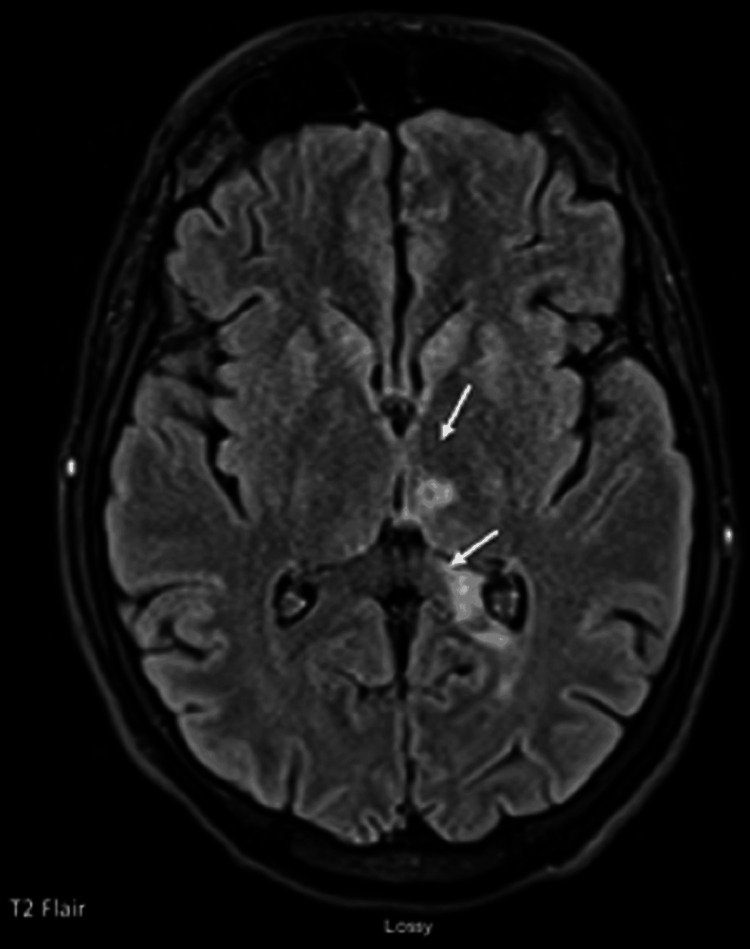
MRI of the head without contrast showing multifocal infarctions (white arrows)
